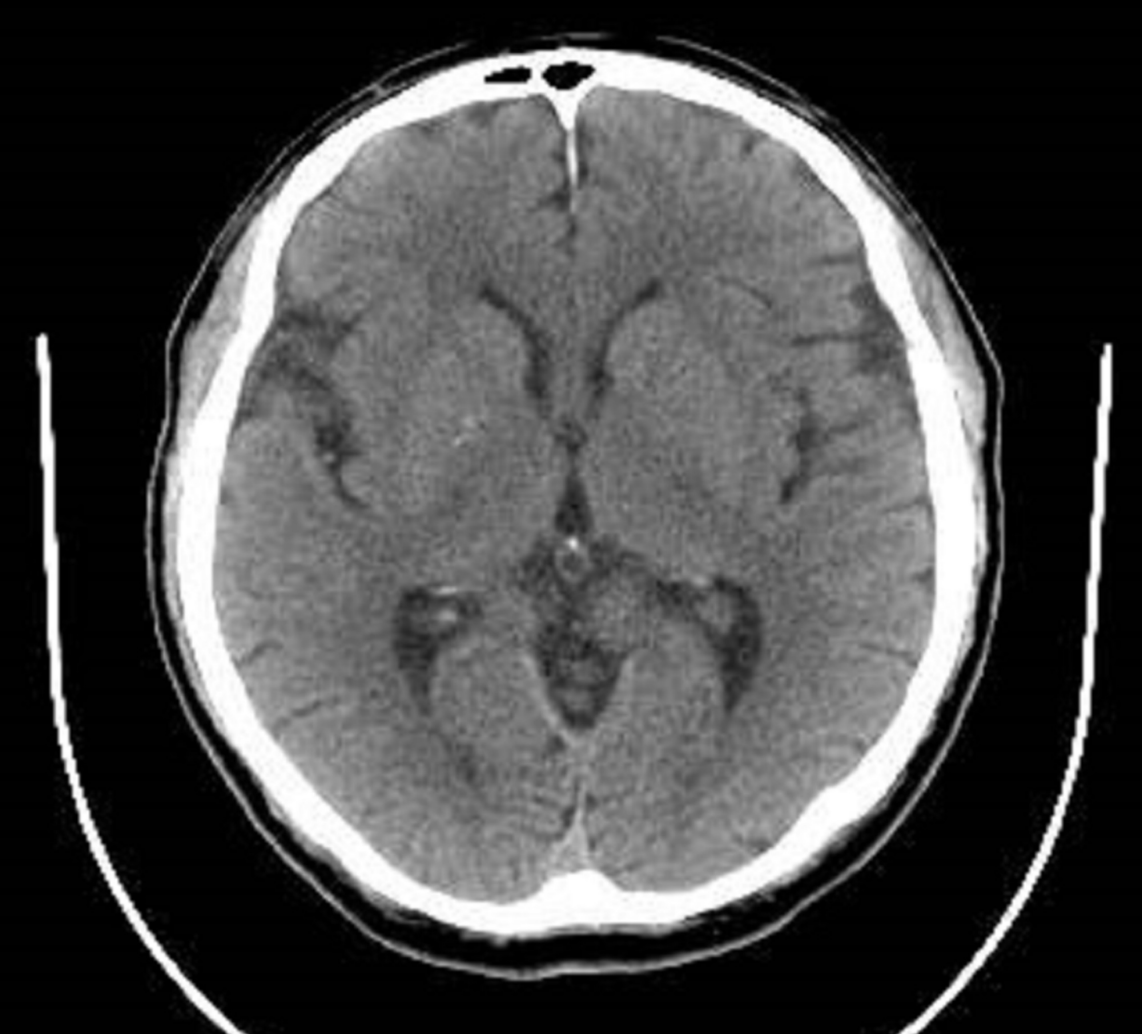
Magnetic resonance imaging of the head
